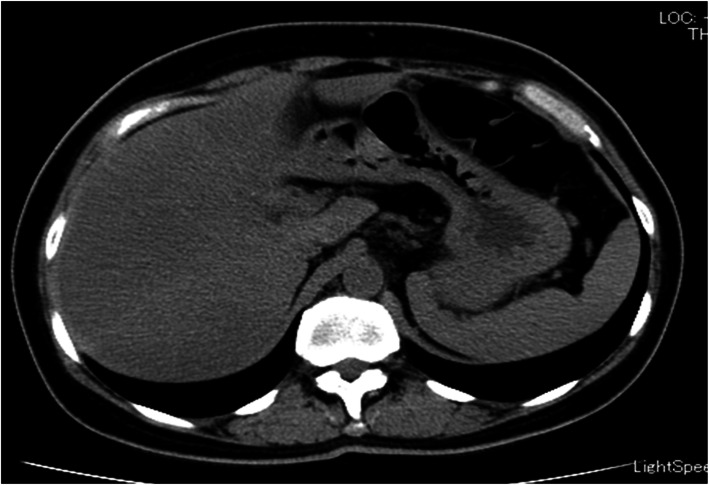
Abdominal computed tomography on admission. The image reveals the presence of fatty liver because the hepatic parenchymal density falls in patchy fashion
